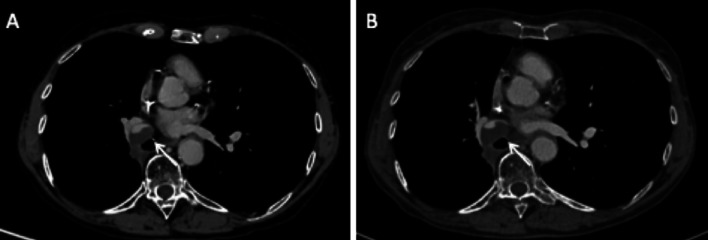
Progressive alluvial thrombus. Axial CT images (mediastinal window) in May 2019 (A) and November 2019 (B), showing an evolutive alluvial thrombus in the interlobar artery stump, respectively, 6 months and 11 months after right lower and middle bilobectomyM.
